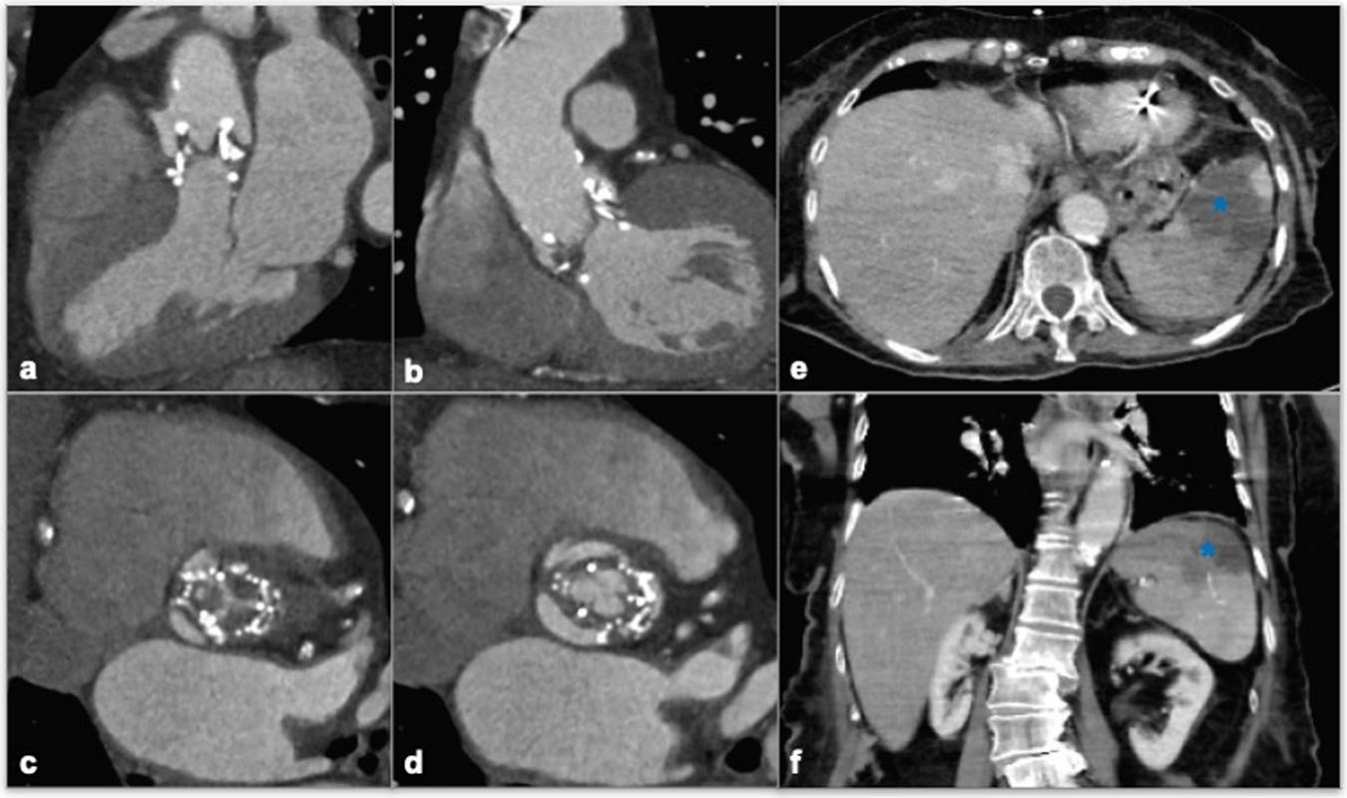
An 85-year-old woman, subjected to TAVI, developing fever and abdominal pain with elevated white blood cell count and C-reactive protein. CCTA is performed in the suspicion of endocarditis and also extended to the abdomen to rule out peripheral embolization. CCTA para-axial, coronal, and sagittal vie (a, b, c, d) show thick ipodense thrombotic apposition over prosthesis leaflets (b, c blue arrow). Abdominal CT also shows splenic infarction due to septic embolization (e, f asterisk). Blood culture turns positive for *Enterococcus faecium*
